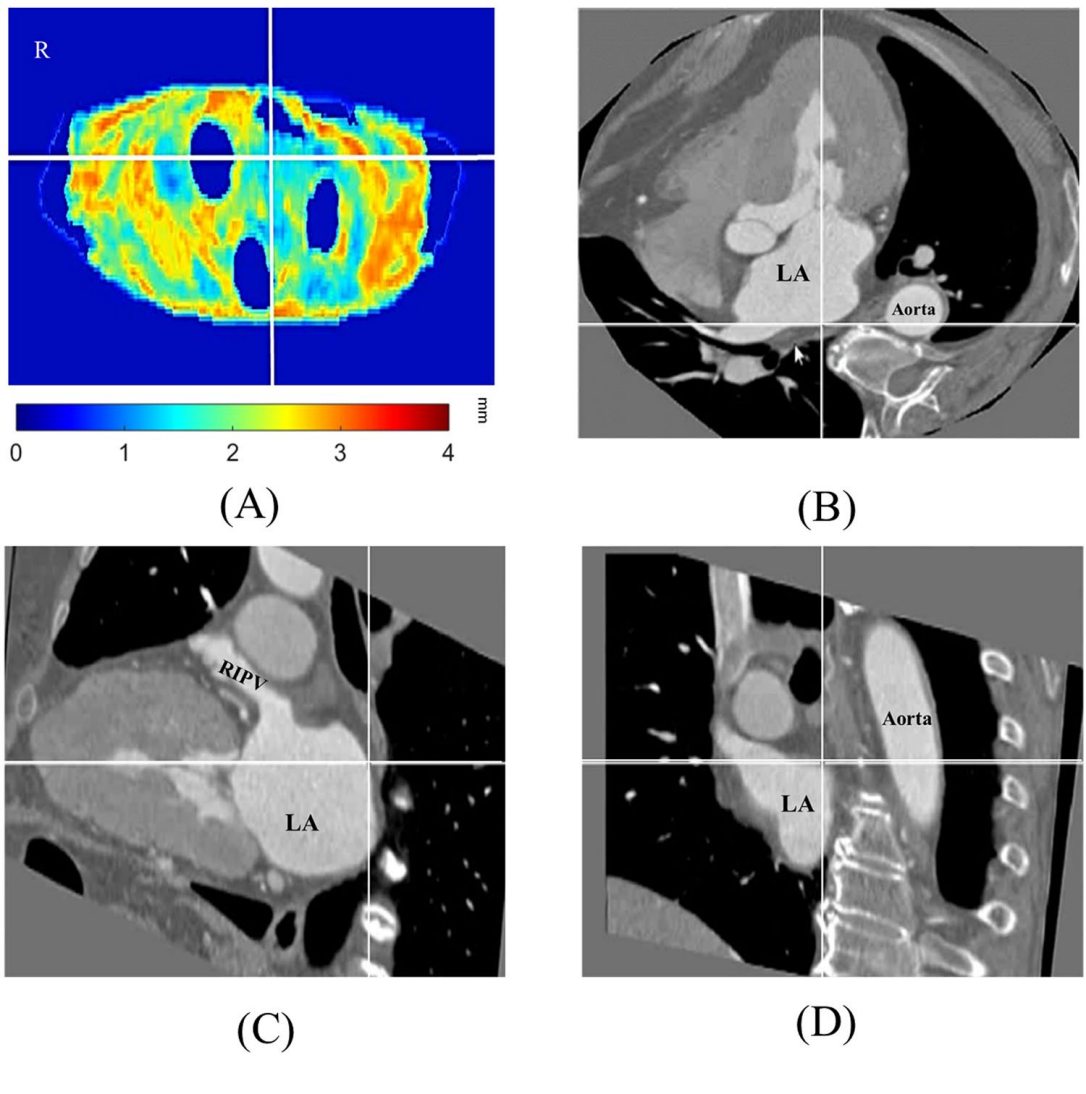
A specific position on the (A) LA wall map can be simultaneously localised on the corresponding cardiac CT images in (B) a 4-chamber view, (C) a 2-chamber view, and (D) a short-axis view. RIPV: right inferior pulmonary vein.
